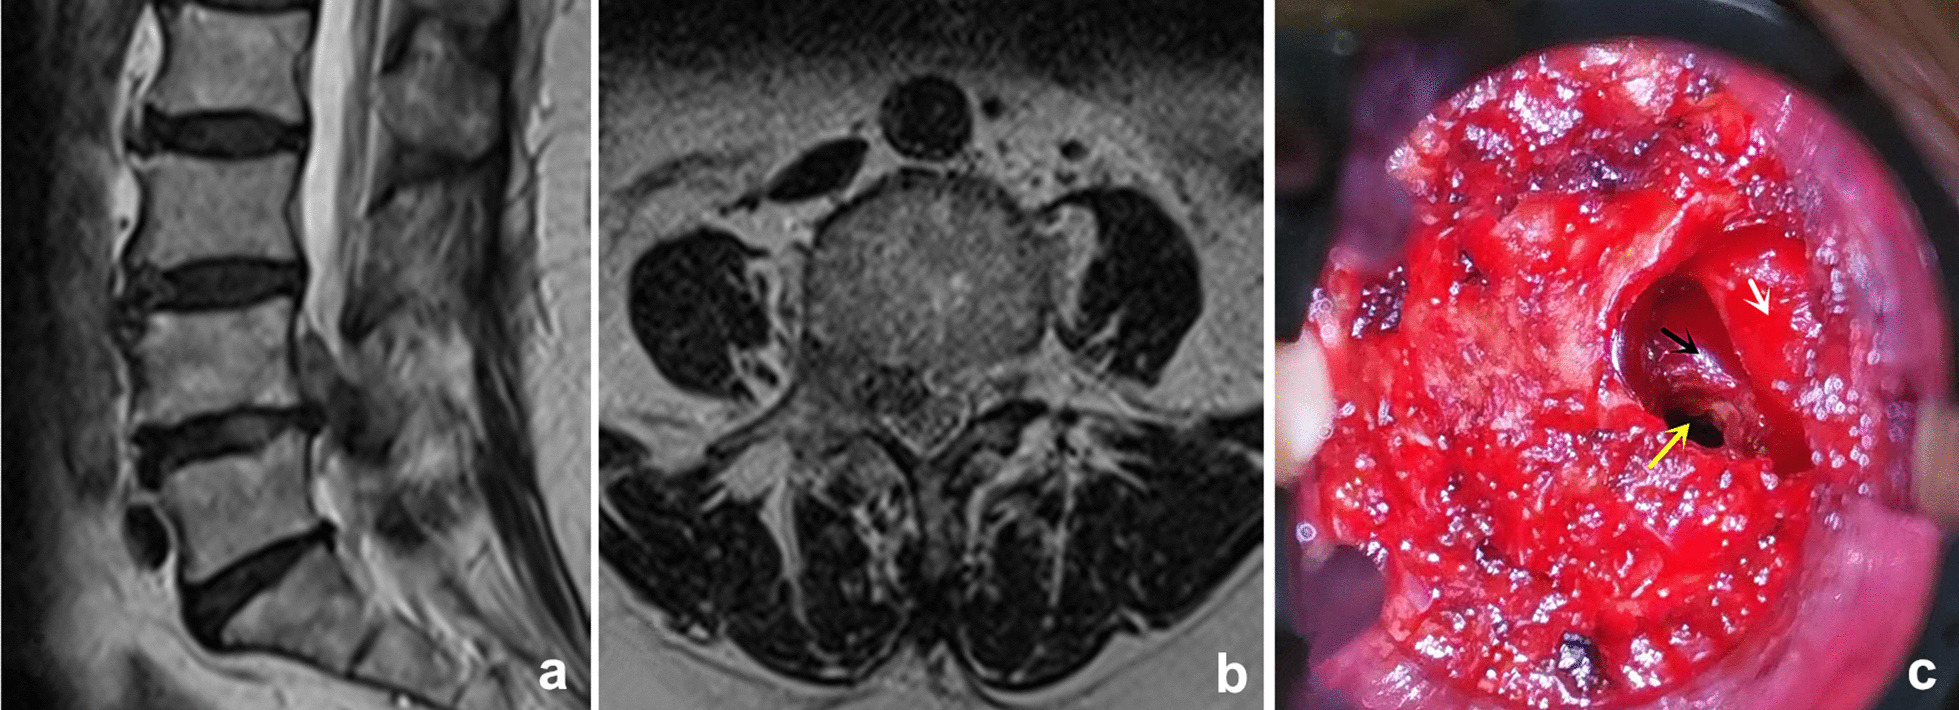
a, b The preoperative MRI showed the migrated fragments were obviously linked with the inferior disc. c The intervertebral space can also be approached after removing a small part of the IAP (white arrow: exiting nerve root; black arrow: posterior wall of vertebral body; yellow arrow: intervertebral space)
